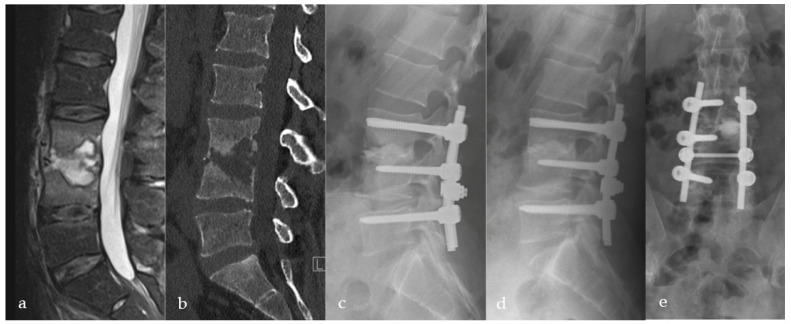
Thirty-eight-year-old male patient suffering from severe erosive spondylodiscitis with a ~40% bony defect in L3/4. Preoperative MRI and CT scans are shown in images (a,b). Immediately postoperatively (c) and one-year postoperatively (d) lateral and (e) a.p. X-rays show bony regeneration and clinical infection consolidation in the affected segment L3/4. L5 was included in the stabilization due to a weak bony situation during the operation to provide sufficient stability.
